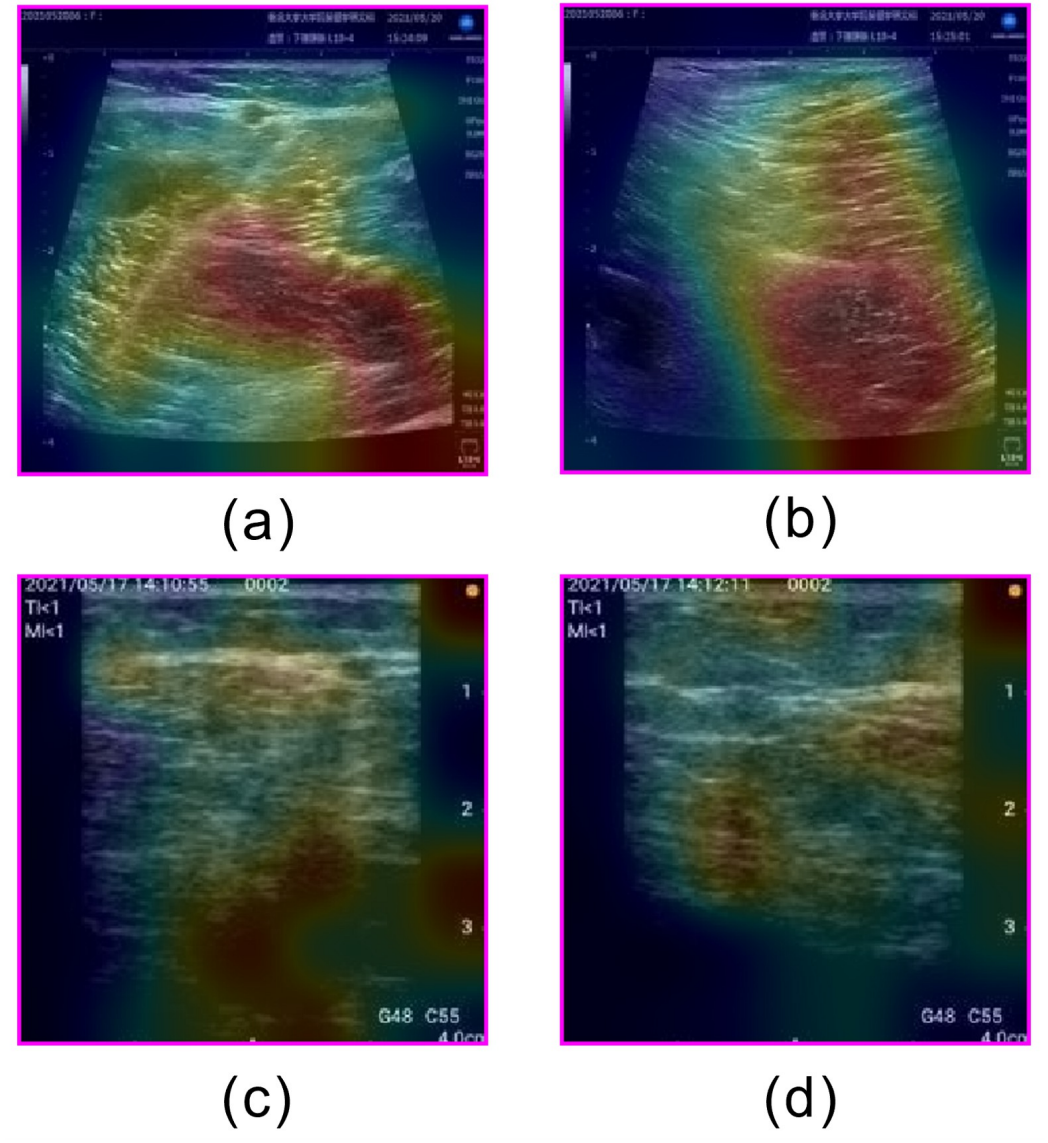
Examples of heatmaps for correctly classified unsatisfactory images. (a) Short axis acquired by stationary ultrasonography equipment, (b) Long axis acquired by stationary ultrasonography equipment, (c) Short axis acquired by portable ultrasonography equipment, and (d) Long axis acquired by portable ultrasonography equipment.
